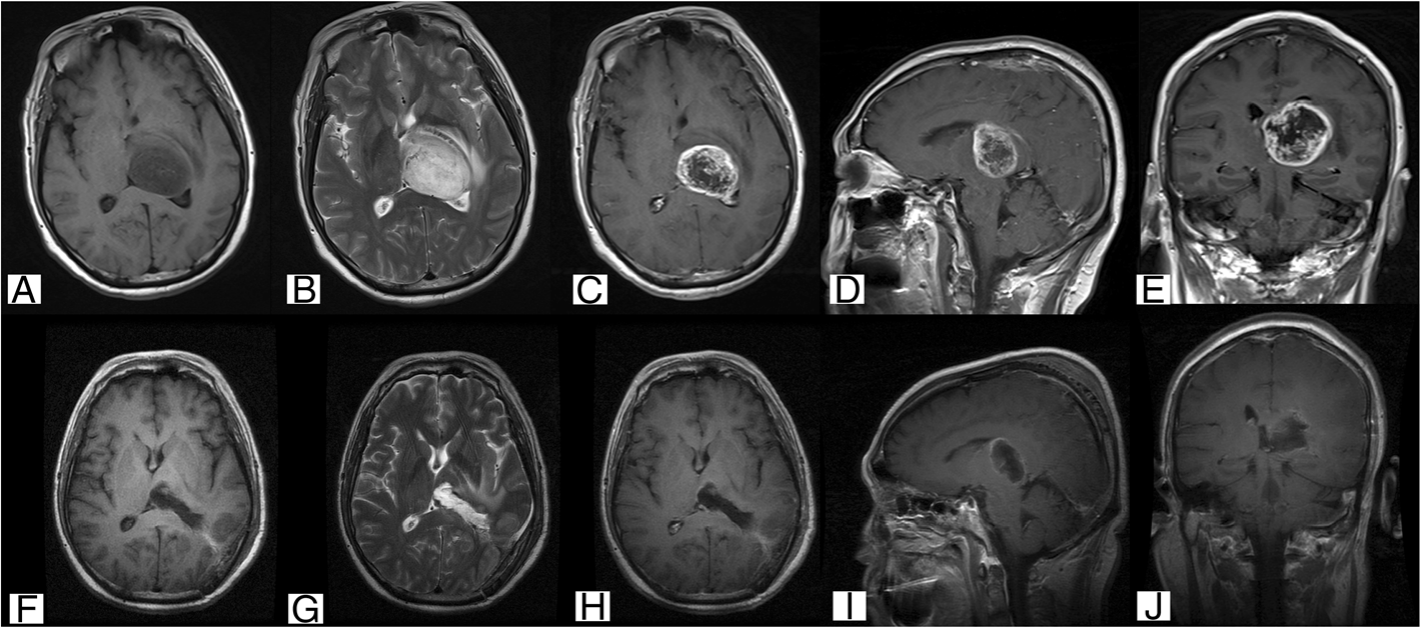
Case 33. Cranial MRI examination revealed unilateral thalamus glioma located in the right thalamus and midbrain with mixed hypointense and hyperintense (a) and hyperintense T2 (b) signals with clear enhancement (c–e). Postoperative MRI confirmed subtotal resection (f–j). Original magnification ×100
